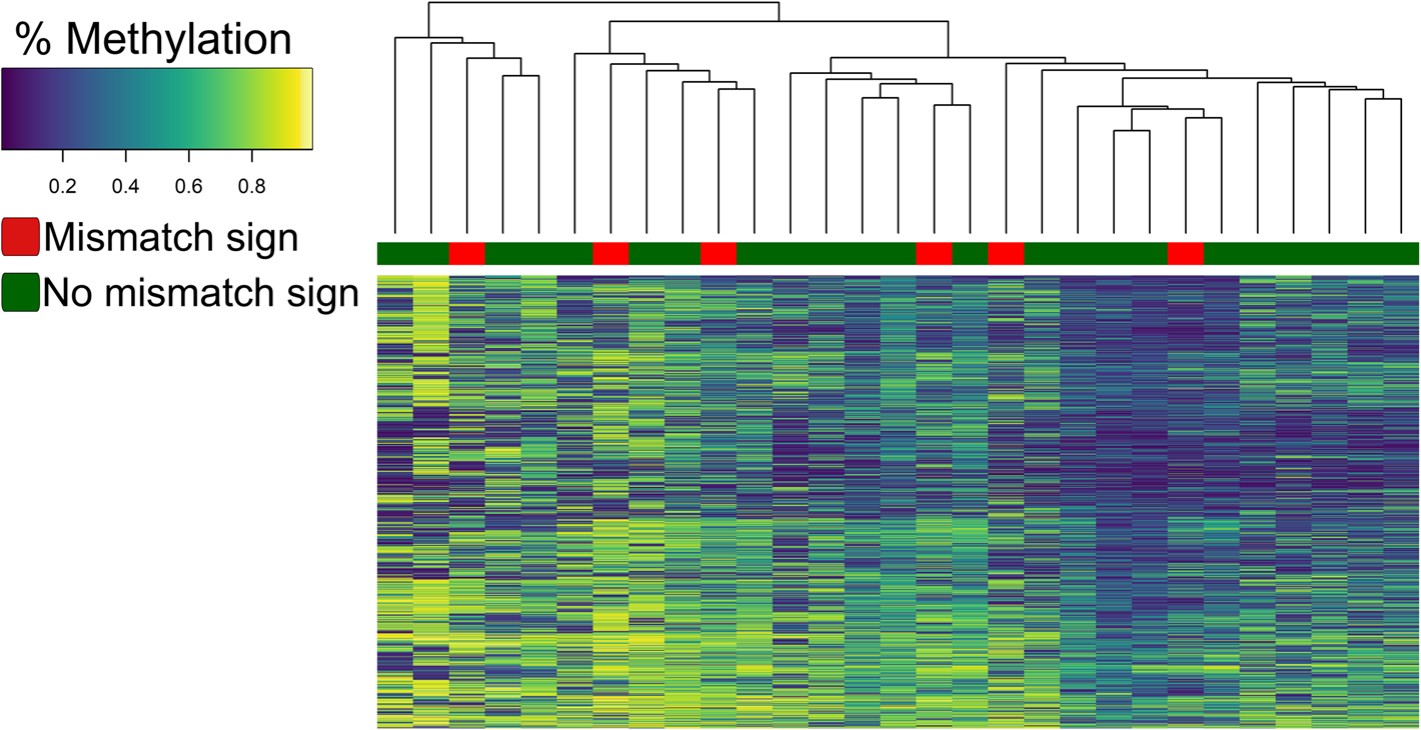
Unsupervised hierarchical clustering analysis on methylation levels for the 5000 most deviating CpG sites in the EPIC methylation array. A value of 0.2 indicated low percentage of methylation and 0,8 a high percentage of methylation. Clustering of the DNA-methylation profiles for the *IDH*-mut astrocytomas in the retrospective cohort (N = 29) did not identify a T2-FLAIR mismatch-methylation associated phenotype
